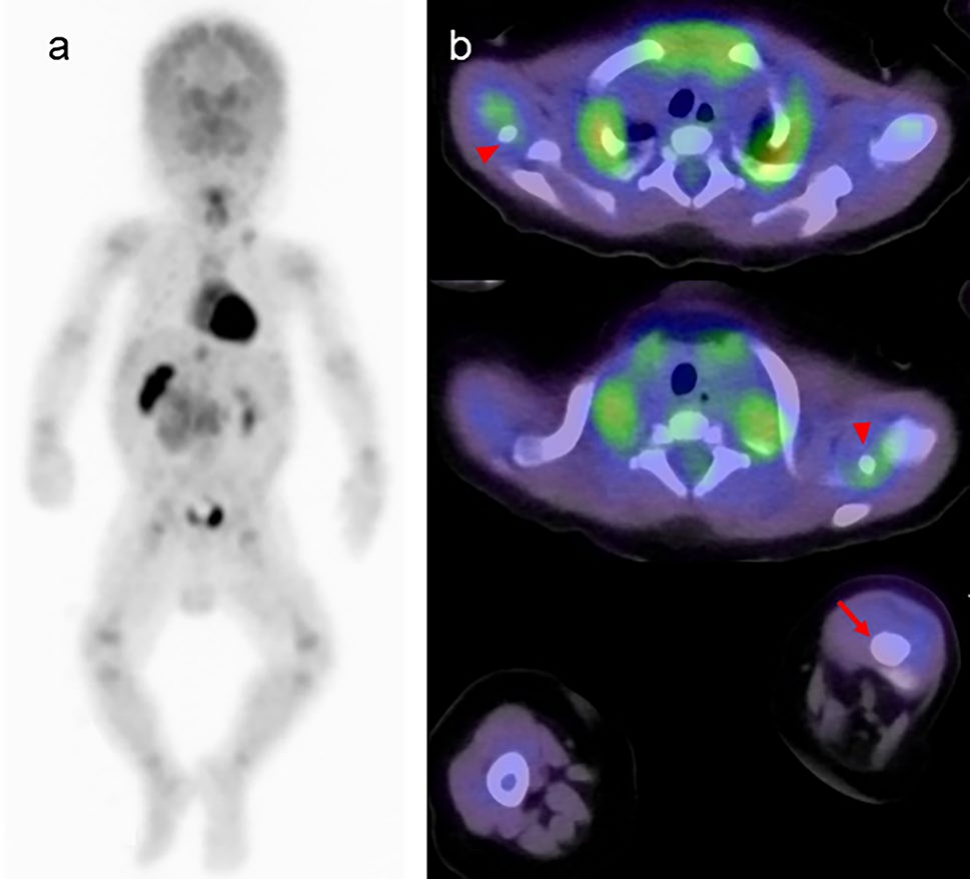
FDG PET/CT image of infants with 31 days after birth. a horizontal band like FDG uptake in the growth plates. b Secondary ossification centers (SOCs) shows slight FDG uptake at the femoral head (arrow head) and no specific FDG uptake at the proximal left tibia (arrow)
